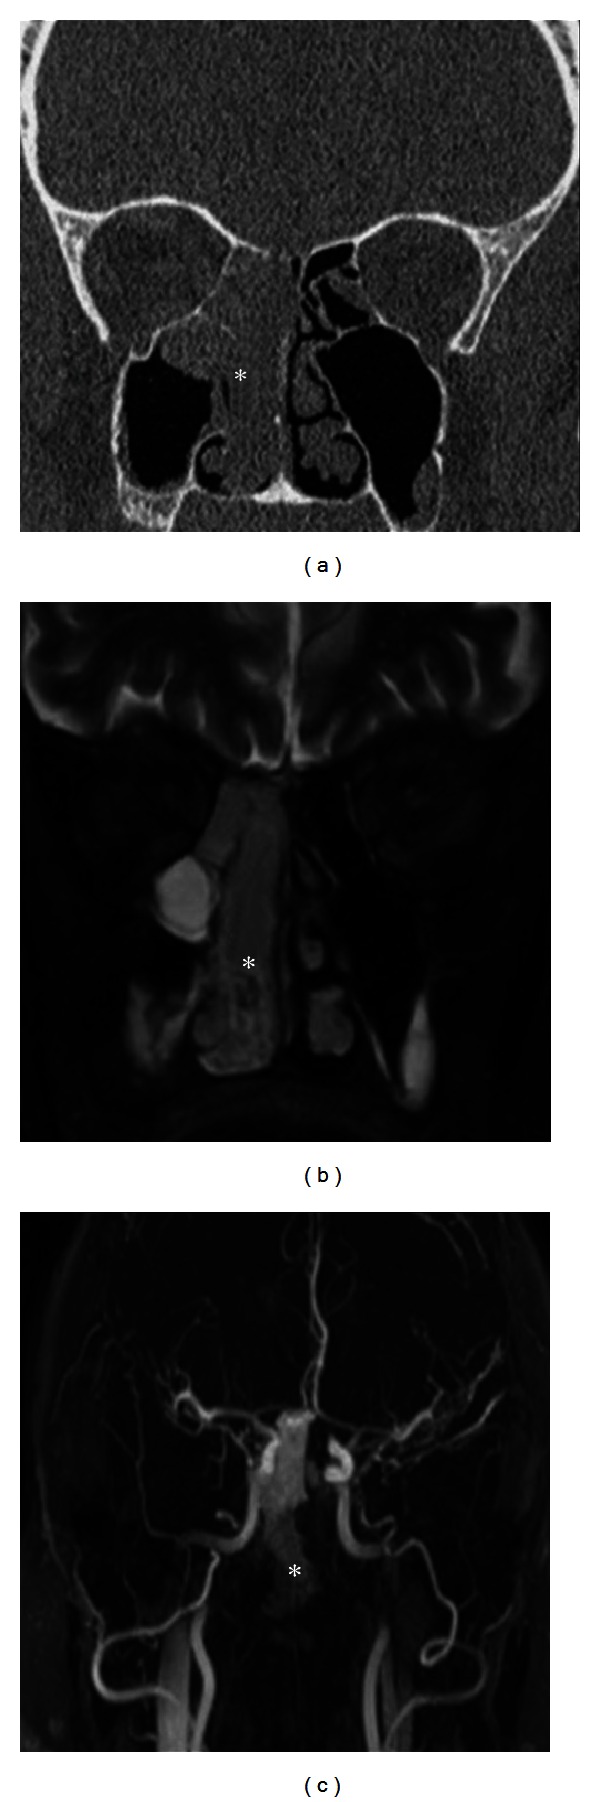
Radiologic findings of sinonasal hemangiopericytoma. CT scan (a), MRI scan (b), and MRI angiography (c). A circumscribed, submucosal, heterogeneous soft-tissue mass measuring 5 × 2.4 × 4.5 cm involving the right dorsal nasal cavity, the right ethmoid, and sphenoid sinus as well as the nasopharynx. A strong enhancement was seen after intravenous contrast administration (*tumor).
